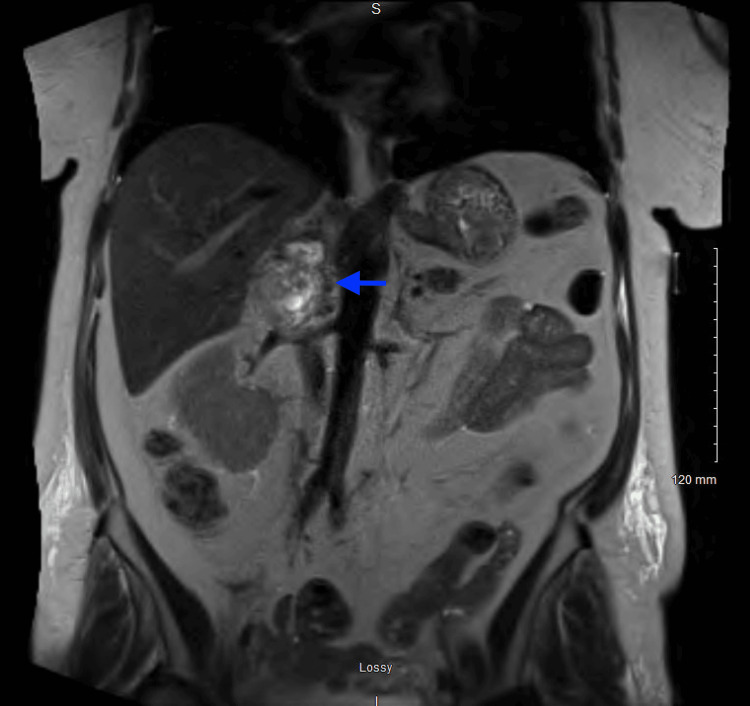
MRI abdomen without contrast - T2 HASTE series (coronal view) MRI abdomen showing a lobulated, 5.8 x 4.6 x 3.8 cm heterogeneous mass (blue arrow) of the right adrenal gland.
